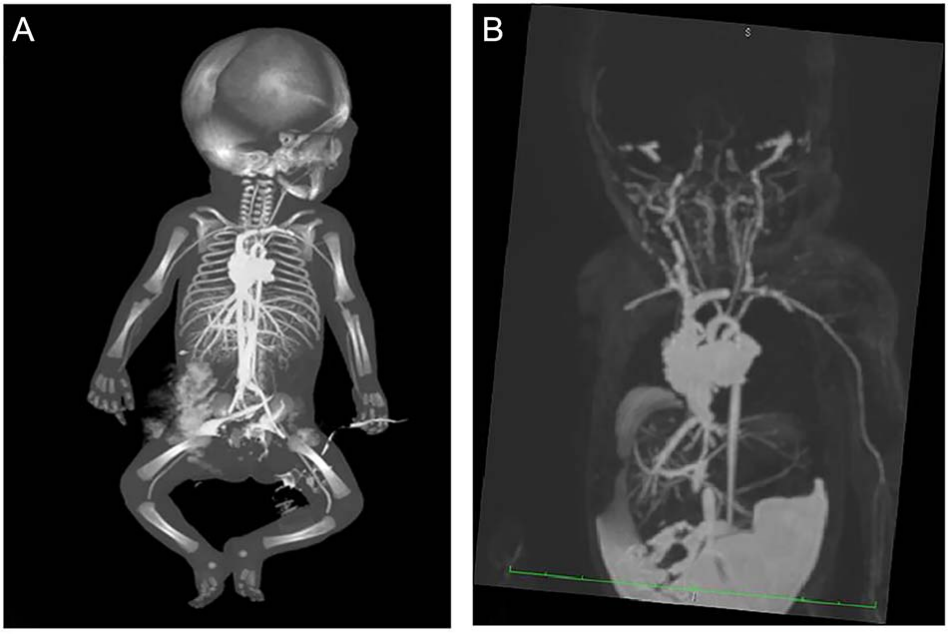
Coronal whole body postmortem computed tomography angiography (PMMRA) (maximum intensity projection reconstructions), general view (A) and zoom (B) showing the absence of signal enhancement of the cervical terminal part of both internal carotids.
